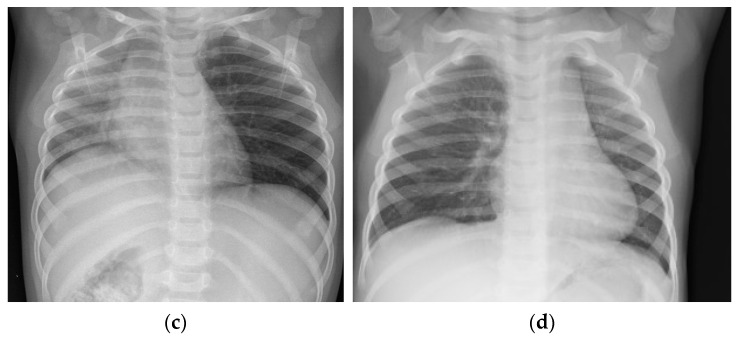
X-ray imaging results. (a) Evidence of foreign body in right bronchi; (b) intensified vascular pattern; (c) mediastinum dislocation to right side; (d) mediastinum dislocation to left side.
